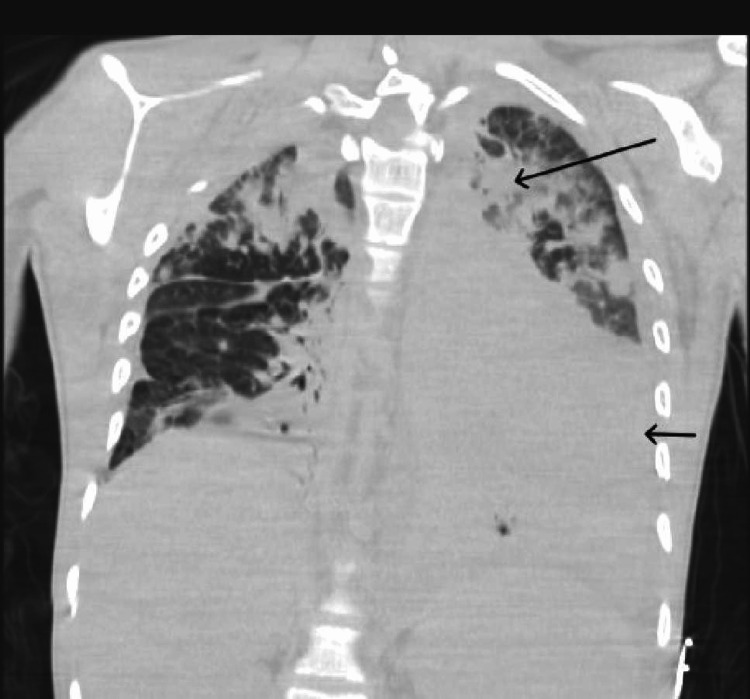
CT chest with IV contrast demonstrating a large left pleural effusion (small arrow) and pulmonary nodules surrounded by ground glass opacities (large arrow) CT: Computed Tomography, IV: Intravenous
